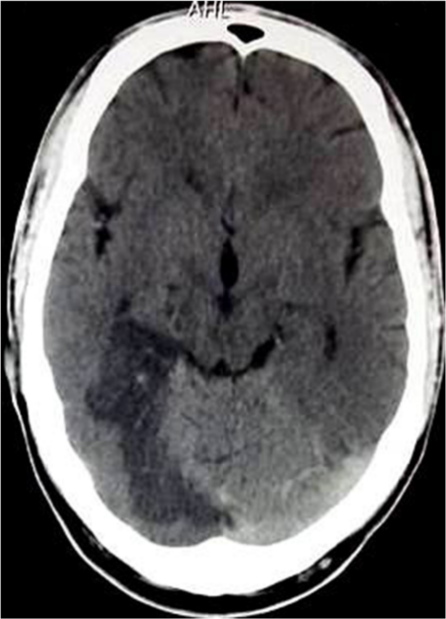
An axial non-contrast head computed tomography showing hypodense area on right occipital lobe consistent with recent posterior cerebral artery infarct
